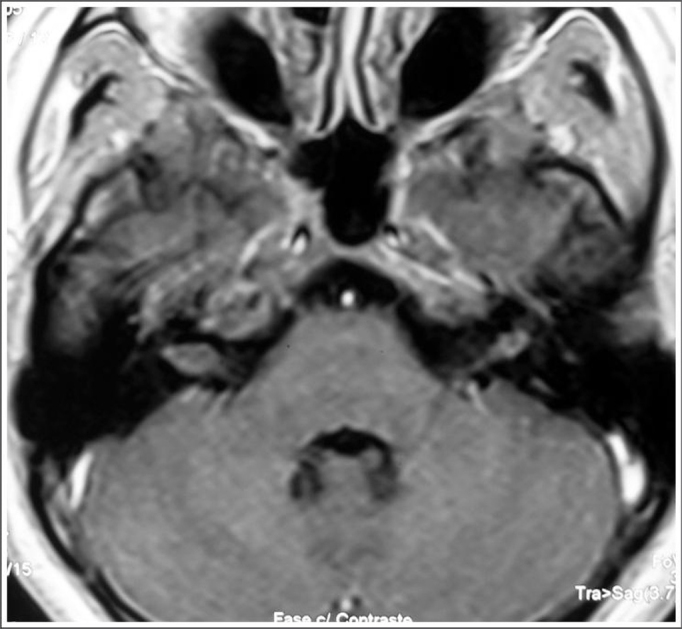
MRI (T1 contrast-enhanced) case 2 preoperative axial view.
